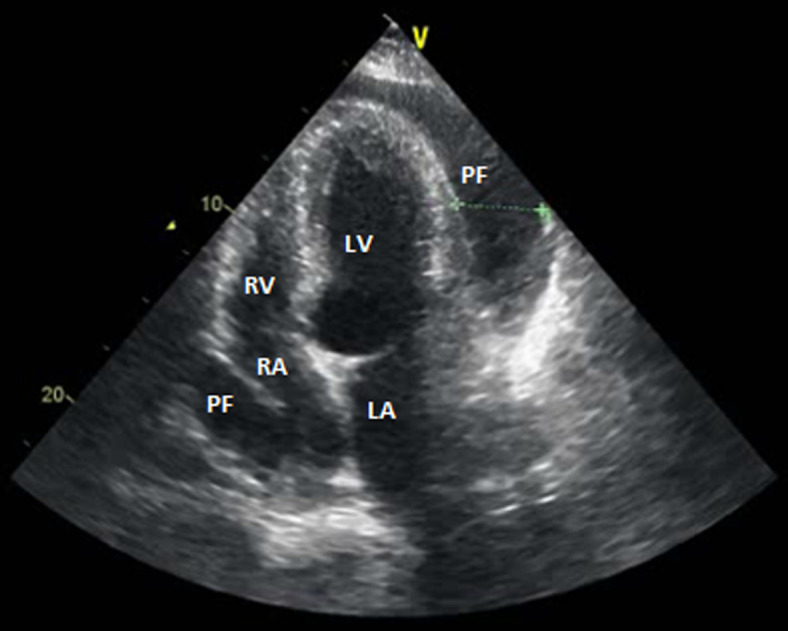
echocardiography apical 4 chamber view showing large pericardial effusion and collapse of the right atrium (RA), RV: right ventricle, LV: left ventricle and PF: pericardial fluid
